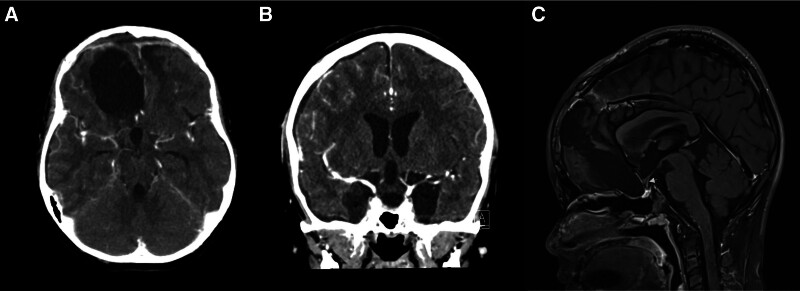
The postoperative radiologic findings and follow-up. Postoperative brain contrast-enhanced CT shows nearly complete removal of the tumor, with preservation of the surrounding parenchyma and neurovascular structures, as seen in the (A) axial and (B) coronal views. During 1-year of follow-up, there was no recurrence with the preservation of the pituitary stalk (yellow arrow) in the (C) sagittal view of MRI. CT = computed tomography, MRI = magnetic resonance imaging.
